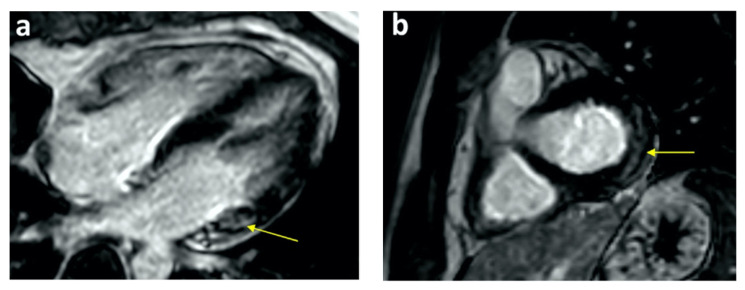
Cardiac MRI PSIR-LGE views show late gadolinium subepicardial enhancement in basal lateral segments in (a) four-chamber and (b) short-axis views. Reprinted/adapted with permission from Ref. [14]. 2021, Elsevier.
